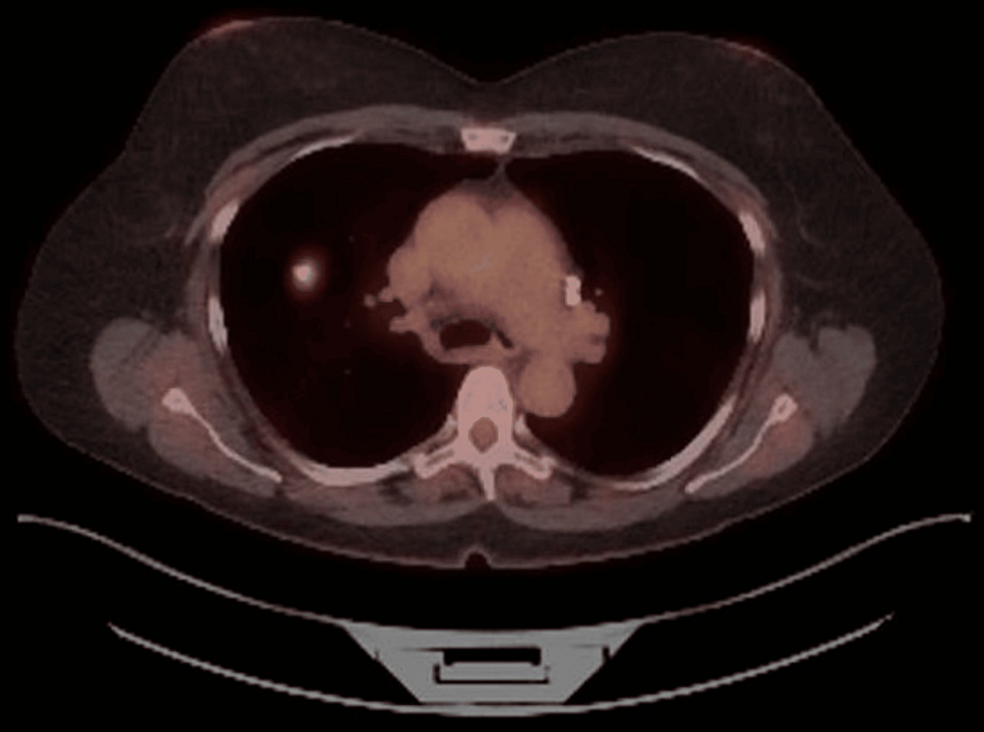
PET-CT scan: hypermetabolic (SUV = 13.1) spiculated RUL pulmonary nodule, consistent with malignancy PET-CT: positron emission tomography-computed tomography, SUV: standard uptake value, RUL: right upper lobe.
